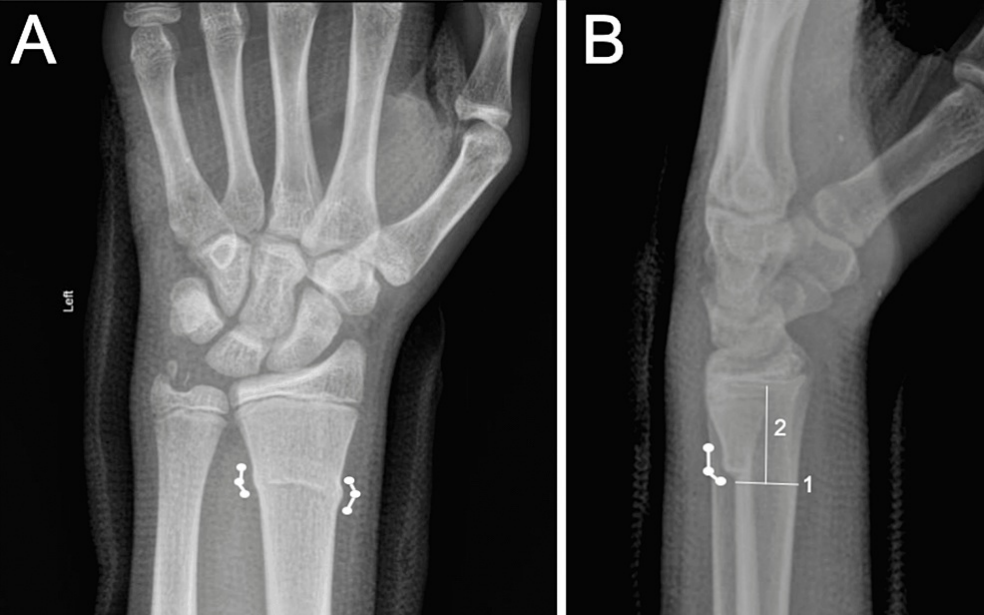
Traditional buckle fracture. Posterior-to-anterior (A) and lateral (B) X-rays of the left wrist in a 13-year-old male demonstrating a DRBF with associated ulnar styloid fracture. (A) Changes in cortical slope are noted as circles along with connecting lines (thick lines) demonstrating the path of the deformed cortical slopes. Note there are two different slopes along the path of the fracture, indicating a traditional DRBF. (B) Thin lines numbered 1 and 2 indicate how the 1-cm rule is determined. Line 1 is drawn at the proximal aspect of the fracture parallel with the distal radial physis (yellow highlight). Line 2 is drawn perpendicular to line 1 and extends to the distal radial physis. According to the rule, if the length of line 2 is <1 cm, the fracture is likely not a buckle fracture and involves the physis. Images courtesy of Dr. MT Niknejad, Radiopaedia.org, rID: 93125 [1].
